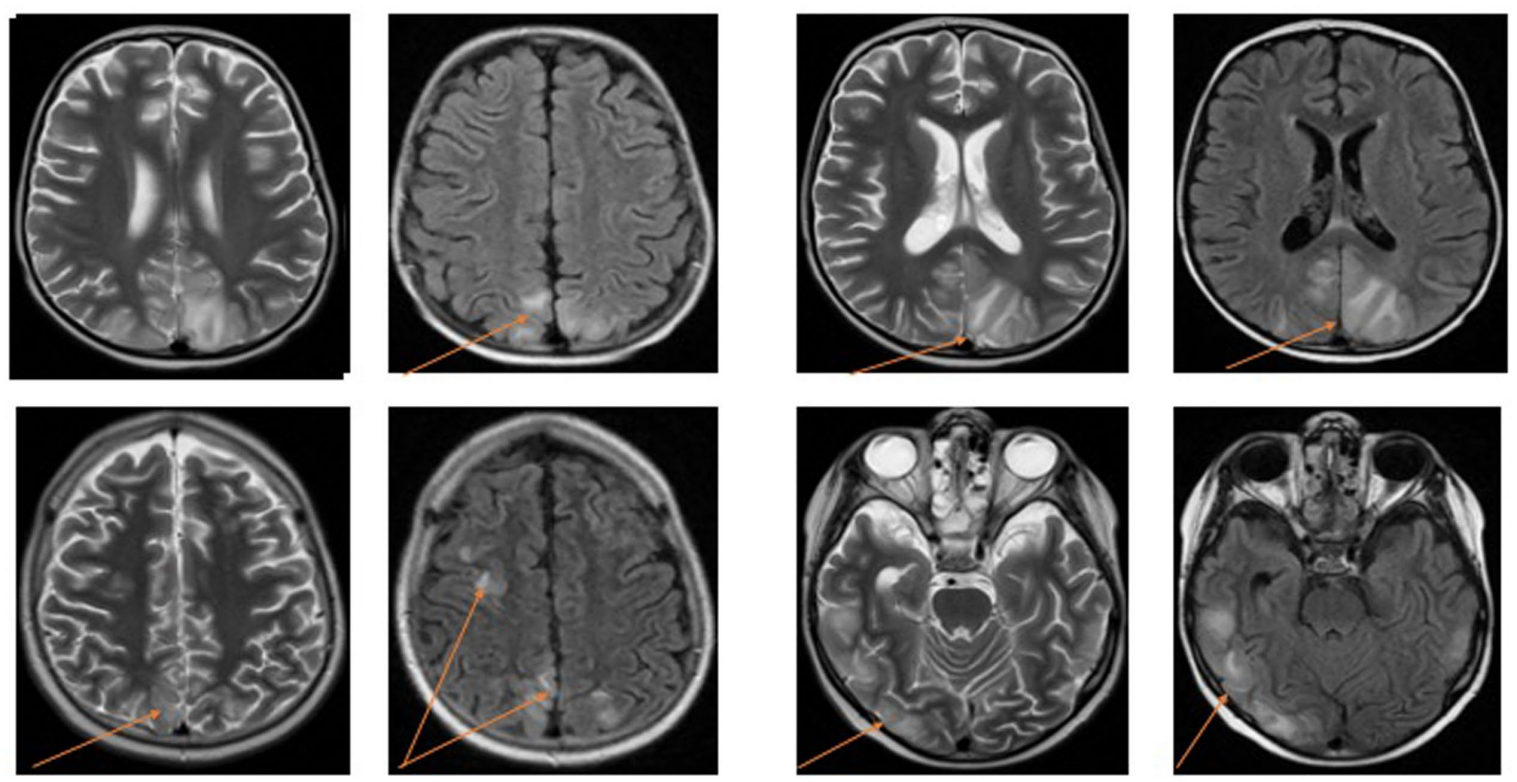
PRES changes in the brain, MRI images from 2 patients.
